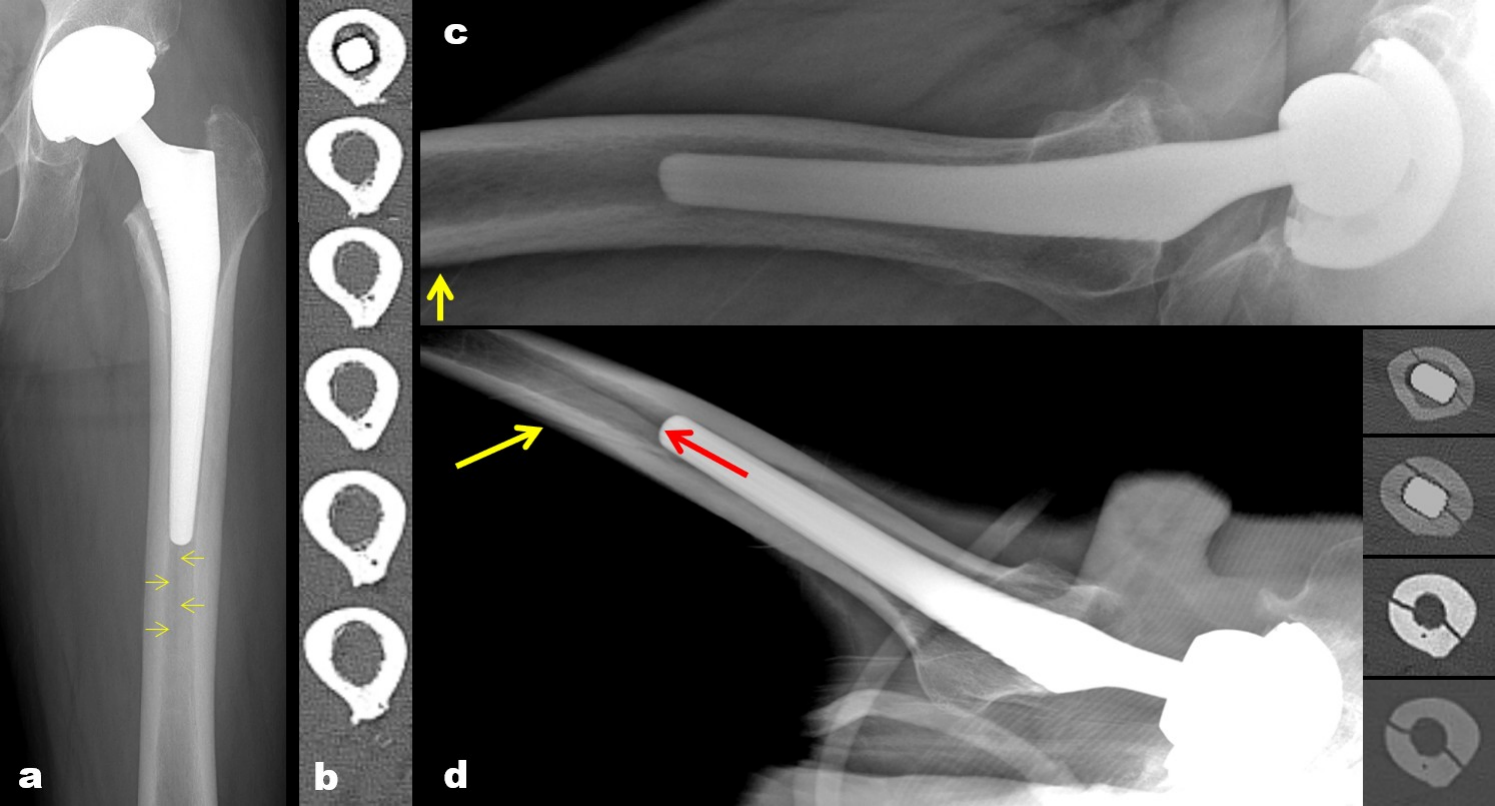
We defined nutrient artery canal of the femur (yellow arrow) as (a) a longitudinal radiolucent line traversing the medullary cavity or (c) an oblique radiolucent line seen traversing the cortex on radiographs. (b) Subsequent axial CT images from proximal to distal show a hypodense line having all of the following three properties in the posterior cortex was accepted as a nutrient canal on CT images: (1) an outer ostium on the outer cortical surface, (2) an uninterrupted course through the cortex, and (3) an inner ostium opening to the medullary cavity on the inner surface of the cortex. (d) We were able to confirm a fracture line (red arrow) in the axial CT images.
